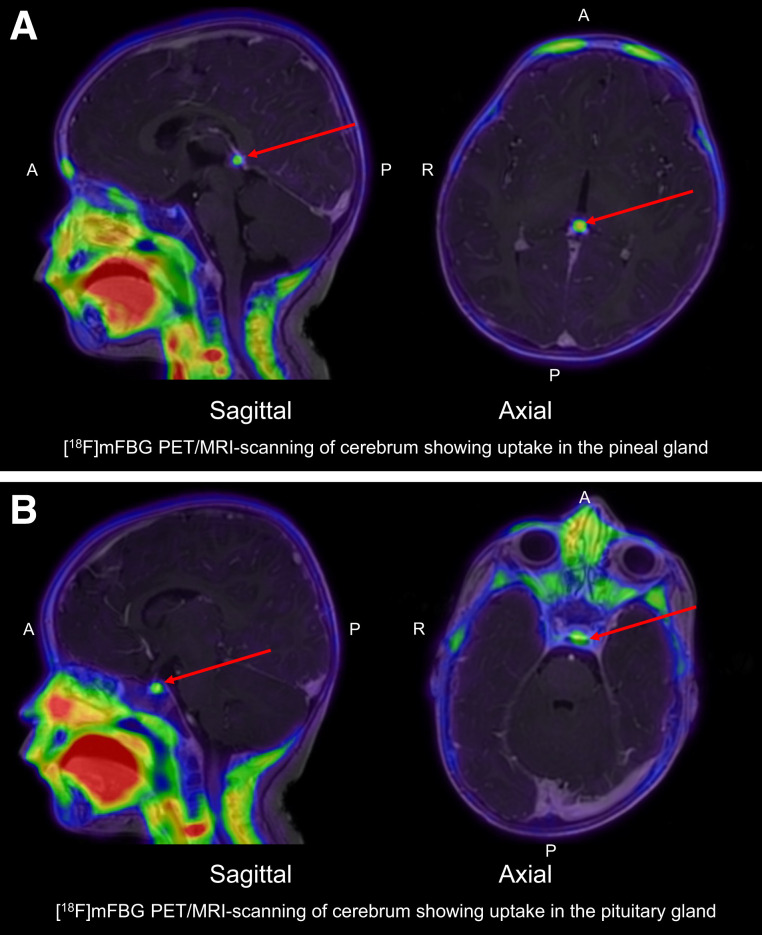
Sagittal and axial view of tracer uptake in pineal gland (arrows, A) and pituitary gland (arrows, B) seen on [^18^F]MFBG LAFOV PET/MRI. A = anterior; P = posterior.
